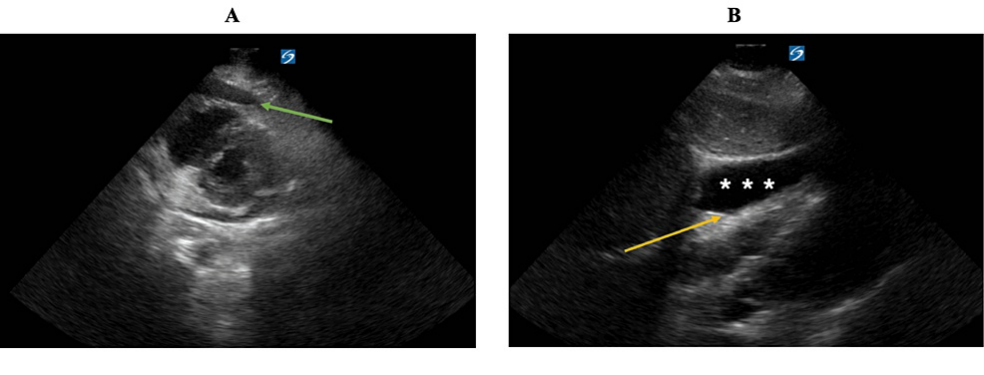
Cardiac ultrasound showcasing pericardial effusion with tamponade physiology A: Cardiac ultrasound with evidence of large pericardial effusion on short axis view (green arrow). B: Cardiac ultrasound with evidence of pericardial effusion (asterisks) and of early diastolic RV collapse consistent with tamponade physiology (yellow arrow) on parasternal long axis view.
